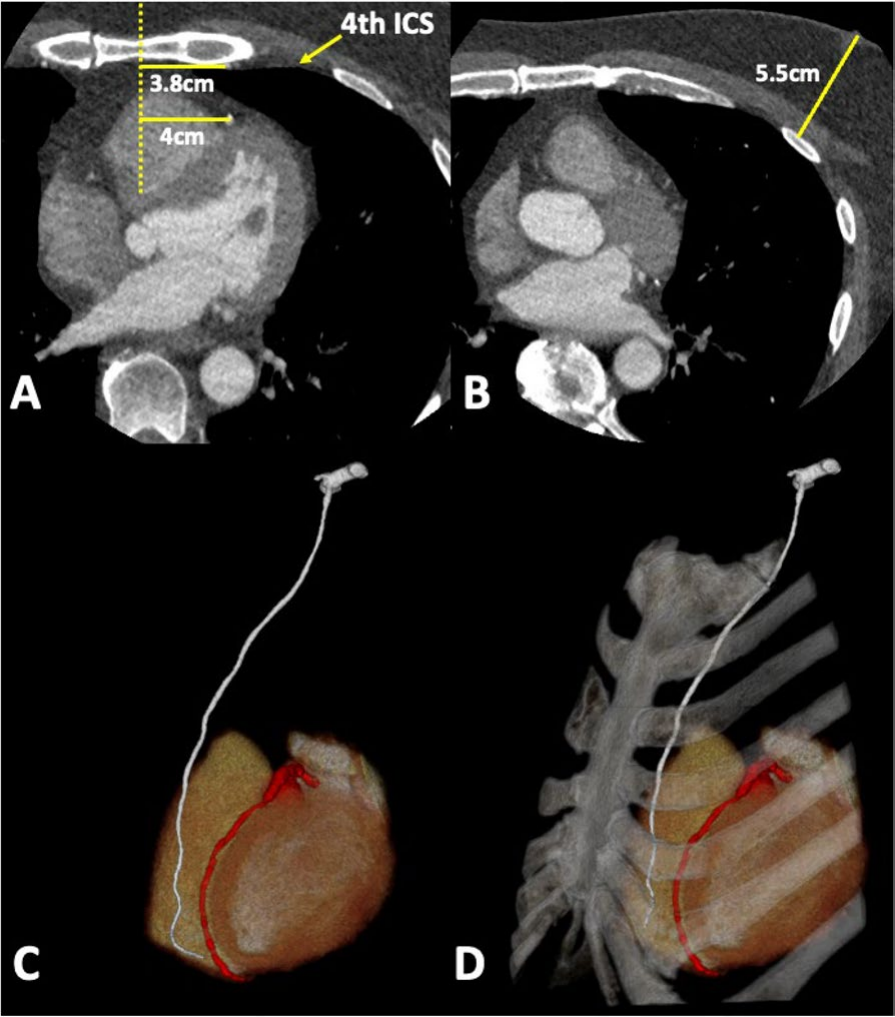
A Axial CT images for patient 9 showing the LIMA to MS (3.8 cm) and LAD to MS (4.0 cm) distances in the 4th ICS, and (B) full field of view axial image at the level of the right nipple showing the NTR (5.5 cm) distance. C Volume rendered image including the LIMA (white) and LAD (red); this is part of the standard 3D visualization, (D) The same view of the volume in (C) including the bony structures for reference. Because the 3D visualization includes individually segmented parts, the display on a 2D screen can be manipulated by the cardiovascular imager and the surgeon
